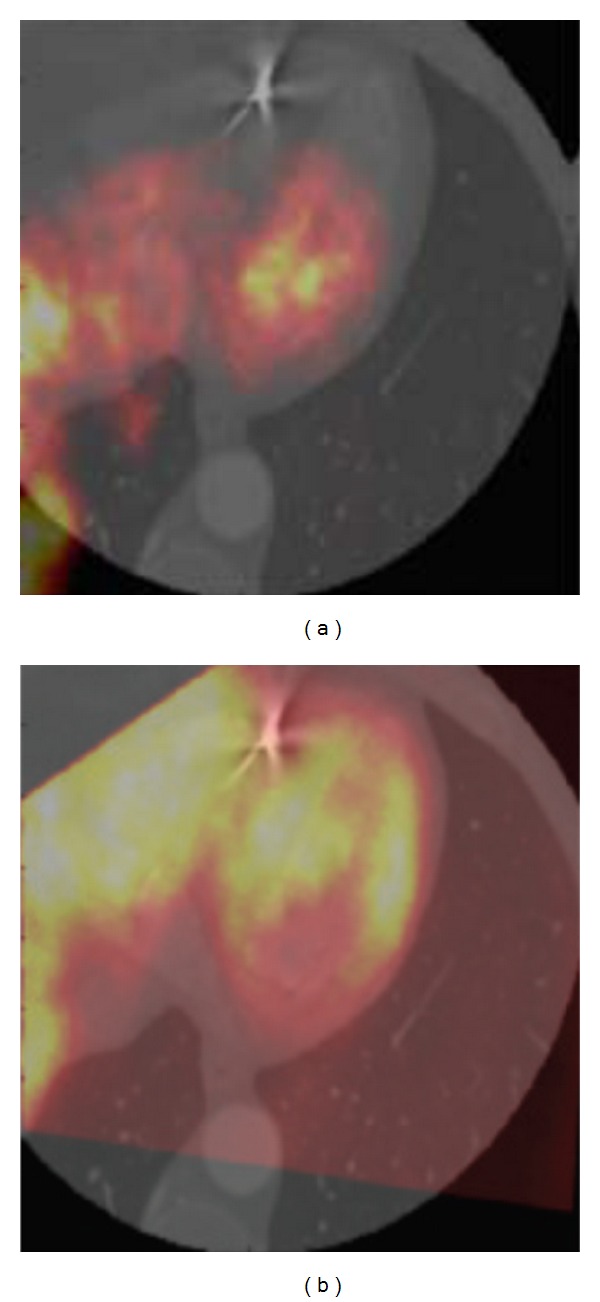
Example of misaligned (a) and aligned (b) transaxial PET and CT images in which an artifact caused by a metallic object is present.
